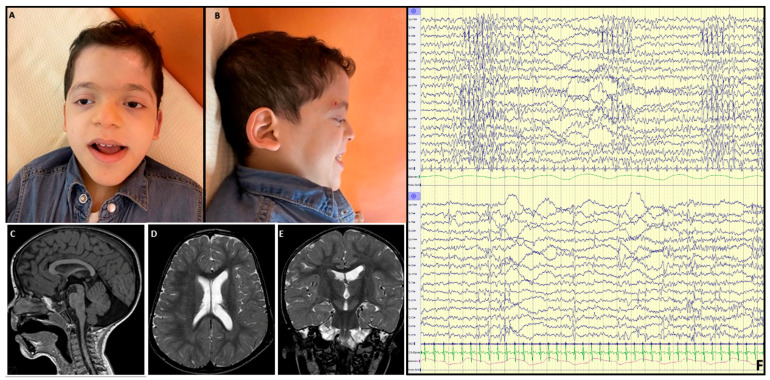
Craniofacial dysmorphism and brain imaging: (A,B) frontal, and side view of proband’s craniofacial dysmorphisms (i.e., coarse facial features, bitemporal narrowing, bilateral frontal and parietal bossing, high forehead, triangular face, synophrys, epicanthus, hypertelorism, bilateral ptosis, depressed nasal bridge, low-set overfolded dysmorphic ears with thick earlobes, long deep philtrum, thick lips, pointed chin). (C–E) A brain MRI was performed at the age of 3. (C) sagittal T1-weighted MRI image shows mild hypoplasia of posterior corpus callosum and mild inferior vermis hypoplasia with enlarged cisterna magna; (D) and (E) respectively, axial and coronal T2w MRI images showing lateral ventricles asymmetry with few dilated perivascular spaces (D) and left incomplete hippocampal inversion (E). (F) EEG shows poor organization with multifocal spike and wave discharges, prevalent in temporo-posterior regions.
